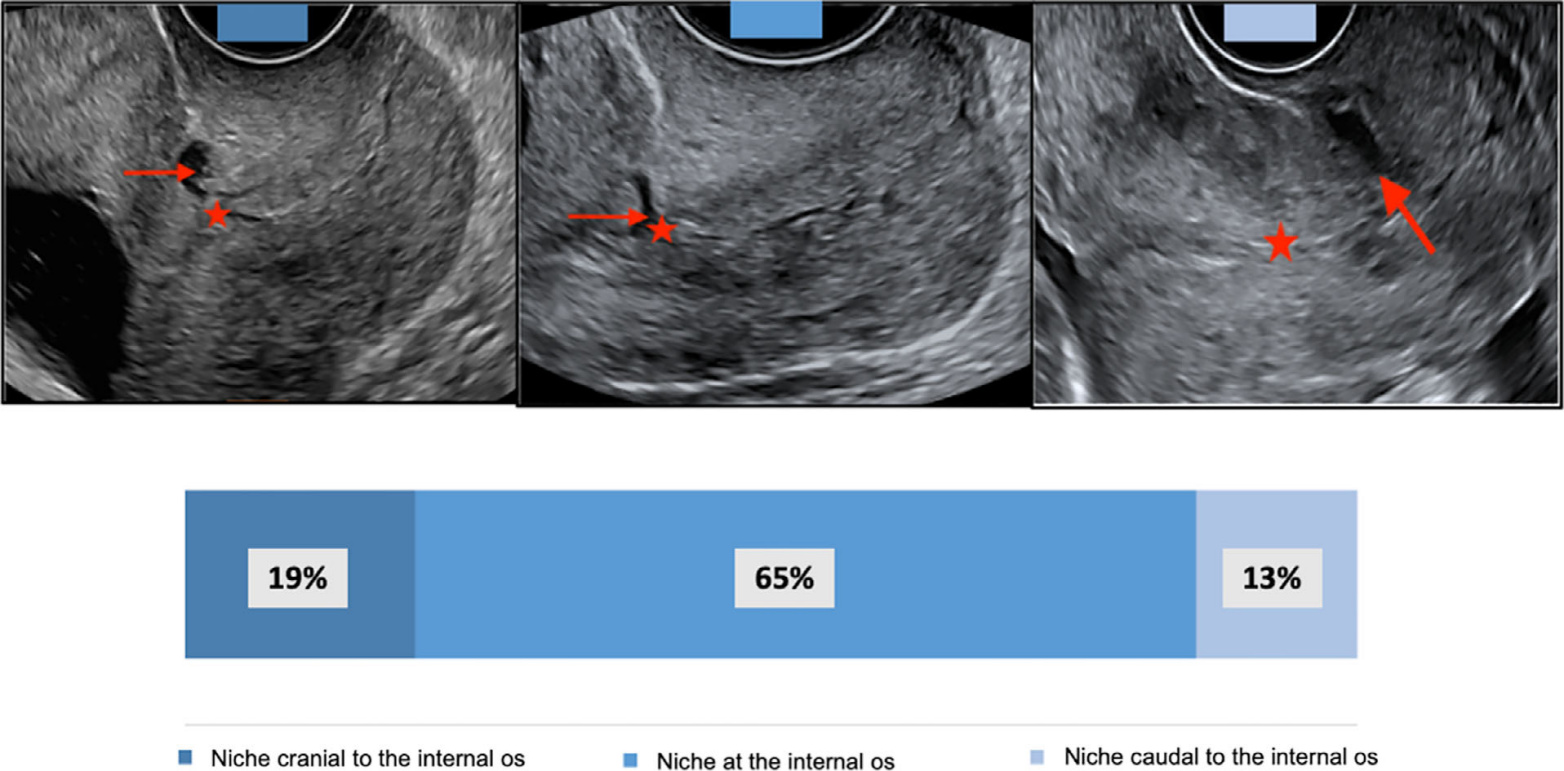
The sonographic position of CB scar niches relative to the level of the internal cervical os (red star: internal cervical os; red arrow: niche).
